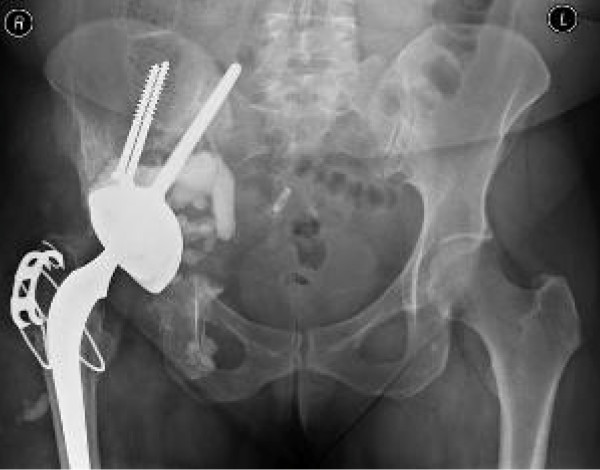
Radiograph one year after revision of the acetabular component.
